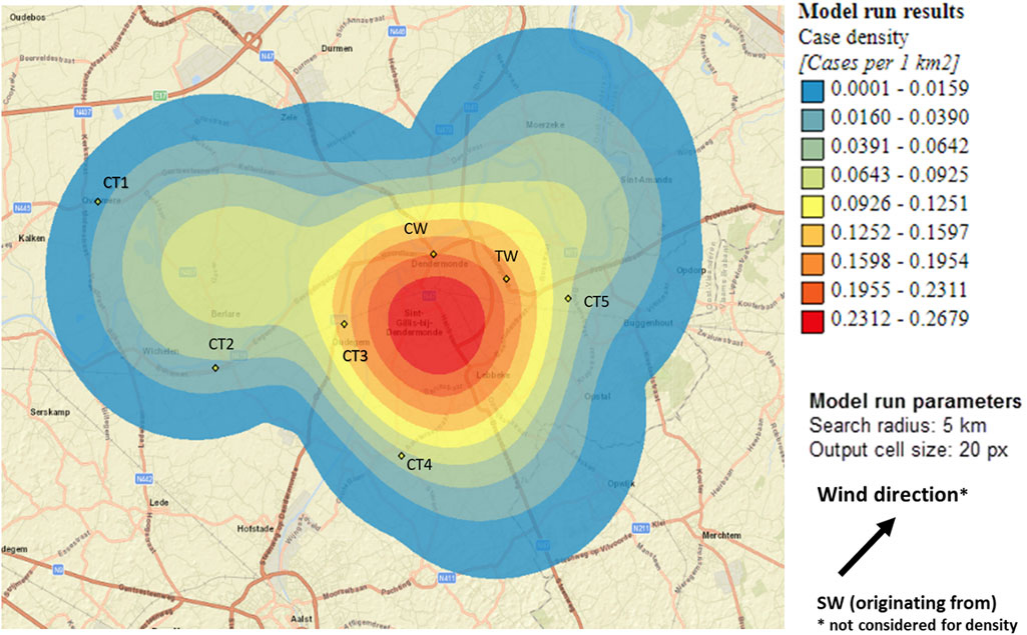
Spatial density of confirmed and probable case residence locations (*n*  =  13) and seven potential source locations CT, cooling tower; CW, car wash; TW, truck wash.
